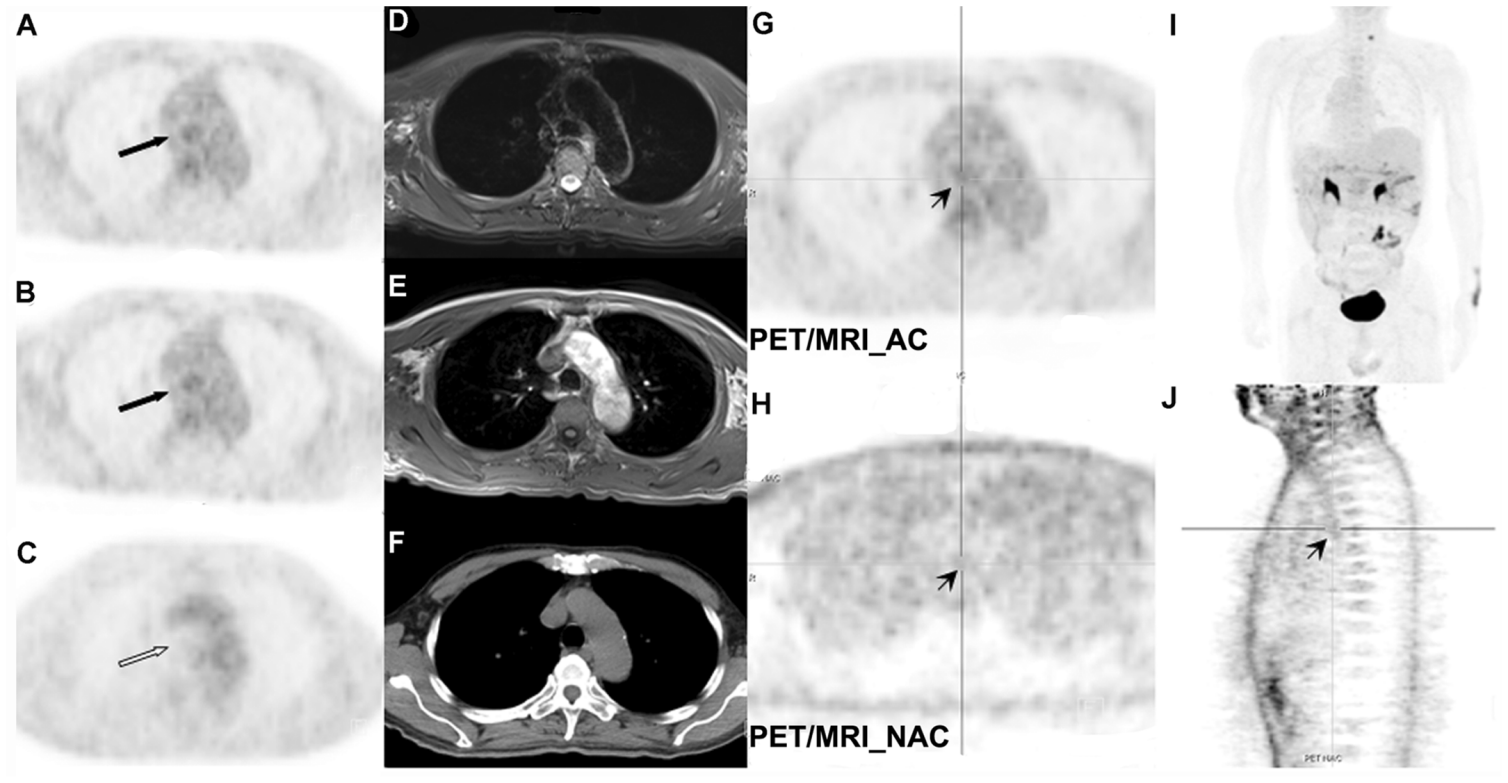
“Hot trachea” artifact on PET/MRI images. The “hot trachea” artifact present on MRI-based FDG-PET images (A and B, *arrow*) compared with CT-based PET image (C). Structural images of T2WI-FS (D), T1WI (E), and CT (F) images showing the corresponding levels of trachea lumen. Although increased radioactivity (*arrow head*) was found in the transaxial MRI-based PET image (G), the maximum intensity projection (I) seemed clear. A similar artifact (*arrow head*) was noted, although less intense, in the non-AC images for the same subject (H and J).
